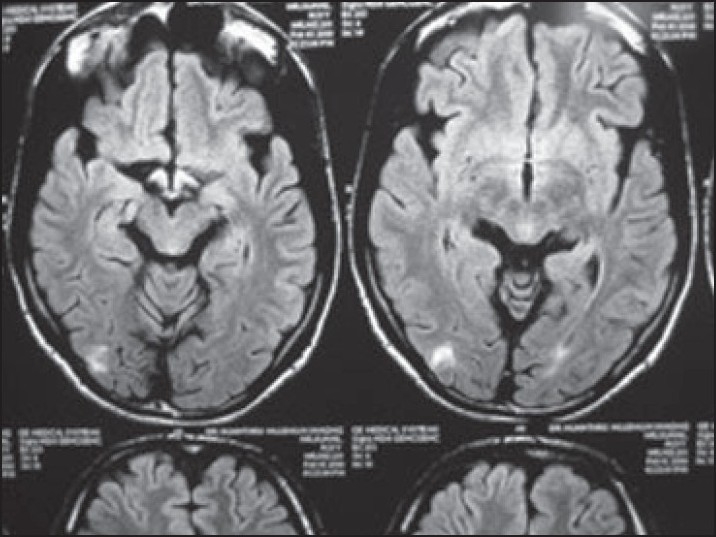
FLAIR image showing a hyperintense signal in the parietooccipital regions; these lesions are typical of PRES.
